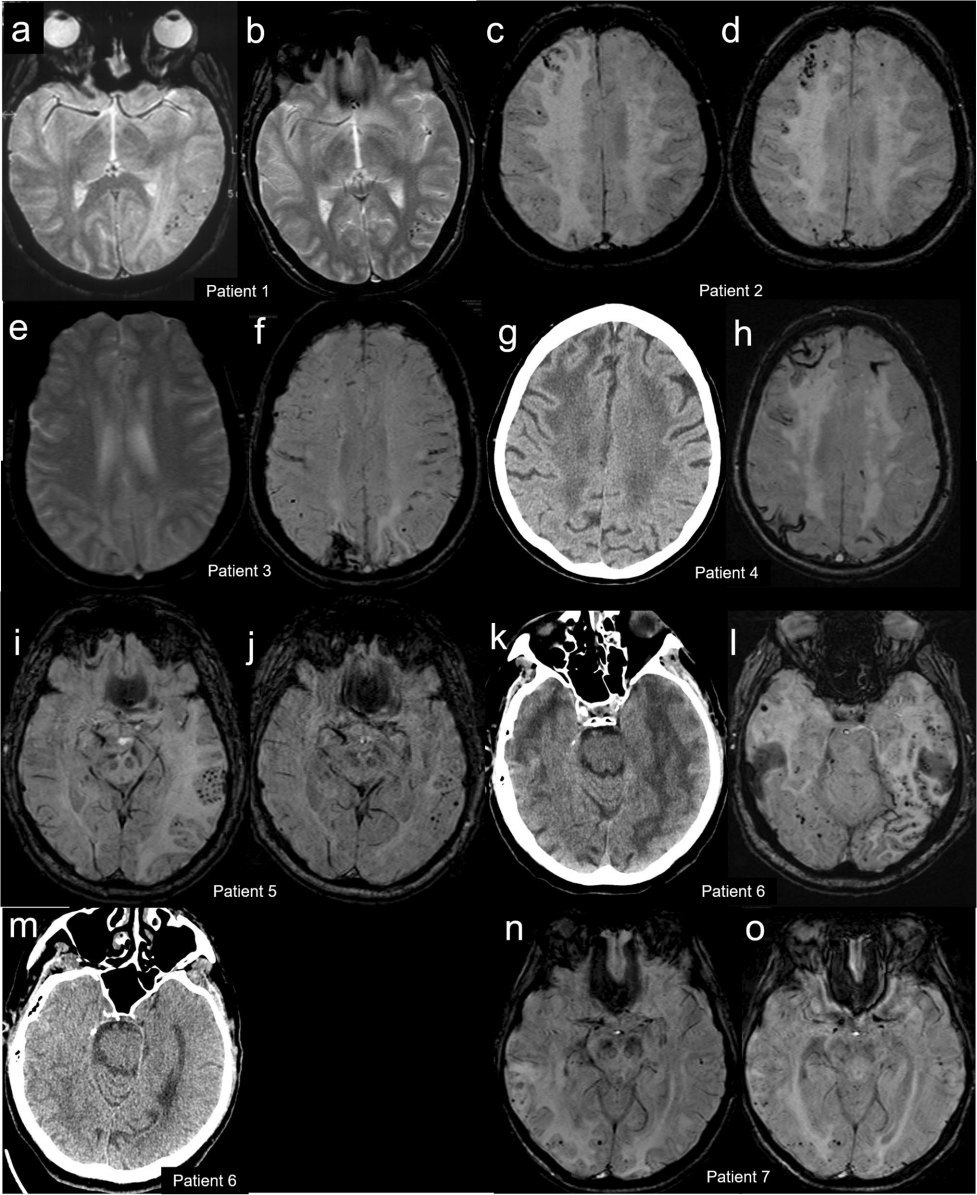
Neuroradiological findings. Patient 1: gradient echo magnetic resonance (MR) axial images at diagnosis (a) and 1.5-month follow-up (b). Patient 2: susceptibility-weighted MR axial images at diagnosis (c) and 1-month follow-up (d). Patient 3: gradient echo (e) and susceptibility-weighted (f) MR axial images at diagnosis. Patient 4: computed tomography (g) and susceptibility-weighted MR (h) axial images at diagnosis. Patient 5: susceptibility-weighted MR axial images at diagnosis (i) and 3-month follow-up (j). Patient 6: computed tomography (k) and susceptibility-weighted MR (l) axial images at diagnosis and computed tomography axial image at 5-month follow-up (m). Patient 7: susceptibility-weighted MR axial images at diagnosis (n) and 1-month follow-up (o)
